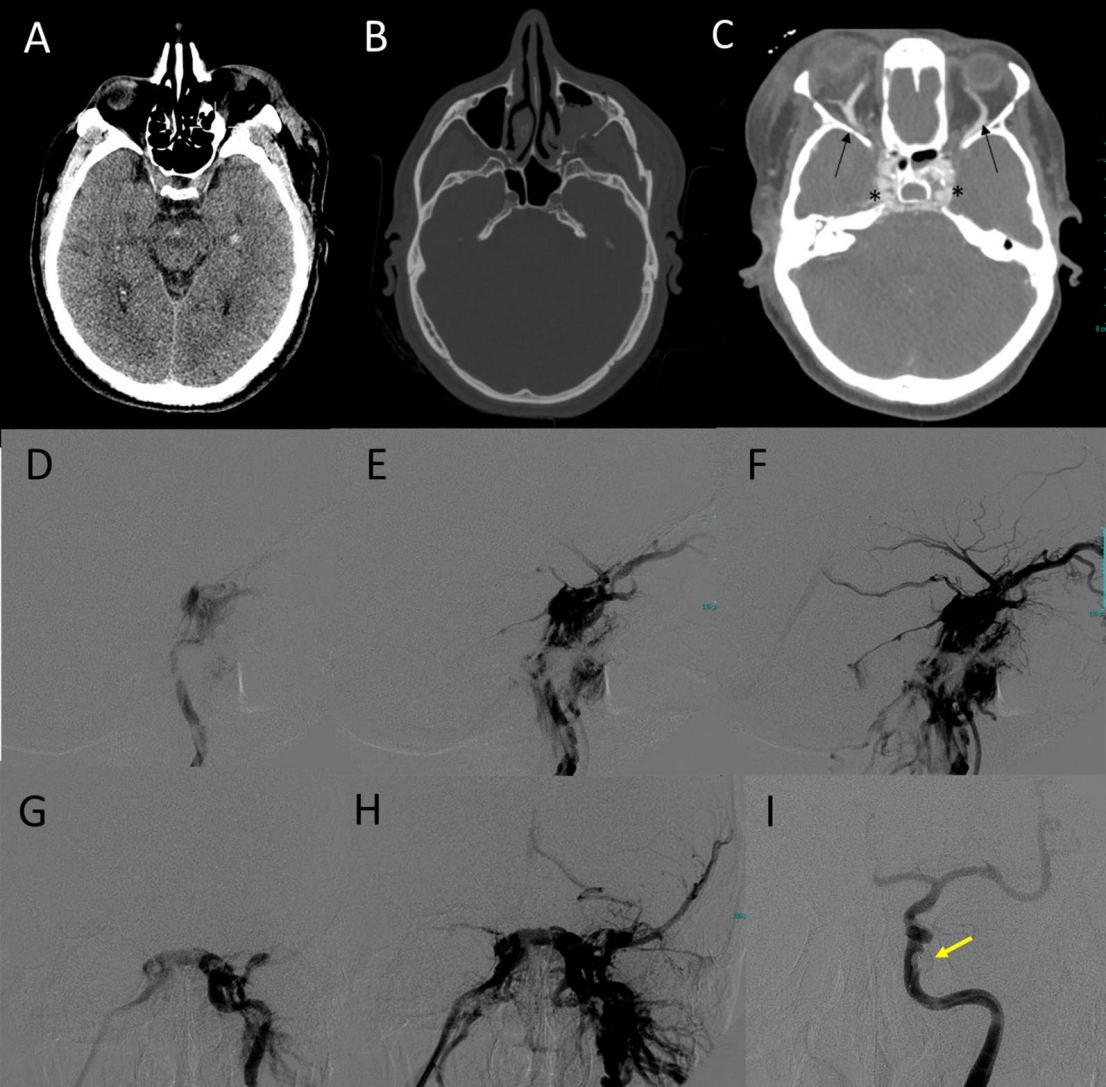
Post-traumatic direct CCF of a patient in the late sixth decade of life following a car accident. Non-enhanced CT scan (A, B) shows the presence of minimal subarachnoid hemorrhage in interpeduncular cistern and a small temporo-mesial intraparenchymal hemorrhage (A), with multiple fractures involving the left zygoma, the left maxillary sinus (B) and the left mandibular angle (not shown). CTA (C) shows prominent opacification and bulging of the cavernous sinus on both sides (asterisks) and bilateral enlargement of the superior ophtalmic veins (arrows). DSA (middle and bottom row) was performed two days later and showed a left CCF (D, E, F: lateral view; G, H: frontal view) with evidence of venous drainage via the anterior route, via the postero-inferior route and with cortical drainage through the left spheno-parietal sinus. Occlusion of the CCF was obtained with a detachable balloon (I, yellow arrow)
